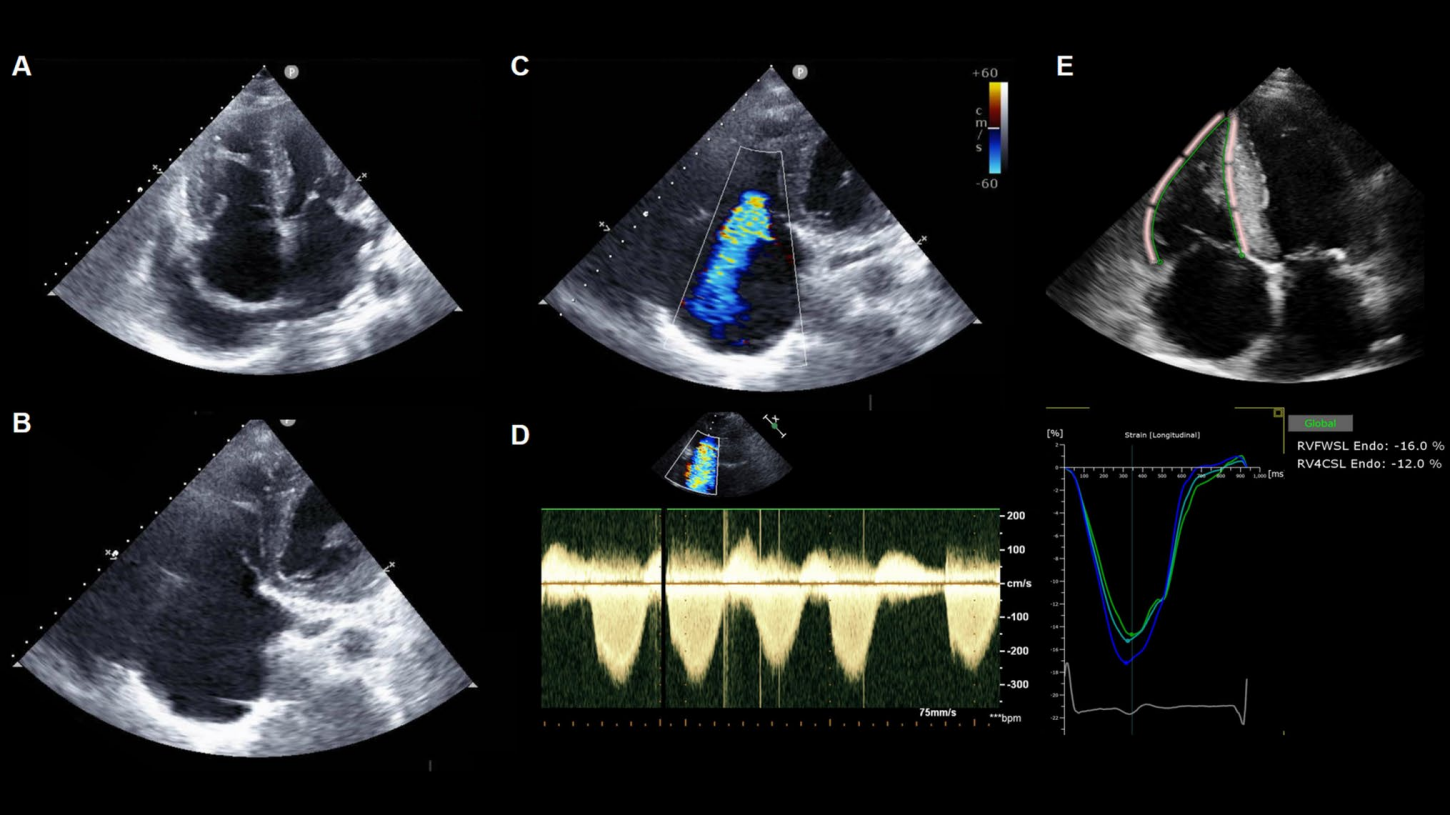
Echocardiographic features of tricuspid regurgitation (TR). Features of TR in an 83-year-old woman with amyloid transthyretin cardiac amyloidosis: severely enlarged right ventricle (RV) and right atrium with retracted septal leaflet (A, B); pericardial effusion (B); massive regurgitation (C, D); depressed systolic RV function (RV free wall strain −16%)
